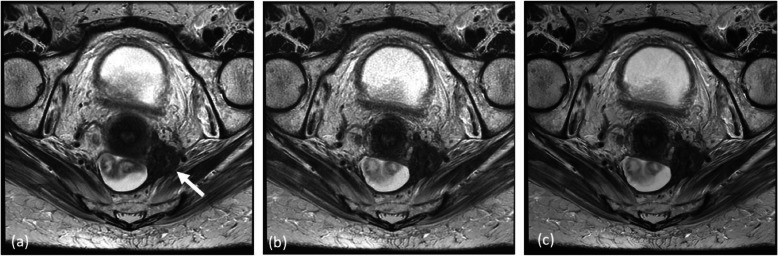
3-T high-resolution axial T2-weighted images of a patient with adenocarcinoma of rectosigmoid junction. (a) Image acquired with a standard clinical protocol with a number of signal excitations (NEX) of 2, with arrow showing a mass with irregular infiltrative margins. (b) Image acquired with an identical scan protocol and patient setup, except with the NEX reduced to 1 and thus scan time reduced by half as compared to (a). An expected reduction in SNR is noted. (c) Image generated from a DL-reconstruction algorithm using the same acquisition as in (b). Notice the restoration of SNR without loss of resolution compared to (b)
